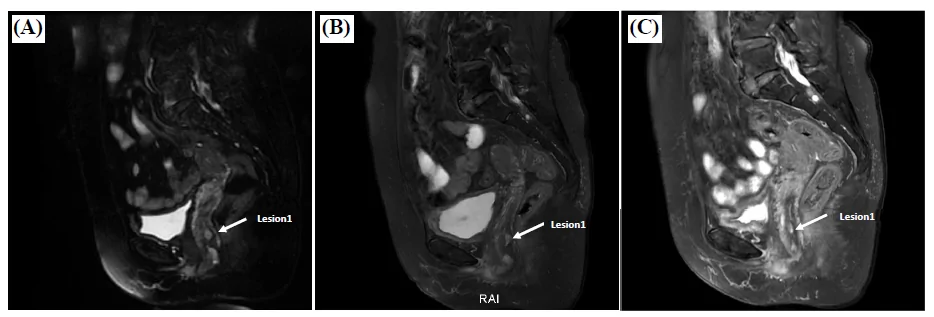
MR Images of the vaginal mass. (A): T2-weighted image of the vaginal Lesion 1 before treatment.TR:4140.0, TE:109.3. (B): T2-weighted image of the vaginal Lesion 1 after external beam radiotherapy.TR:3687.0, TE:70.0. (C): T2-weighted image of the vaginal Lesion 1 six months after treatment. TR:4097.0, TE:67.4.
